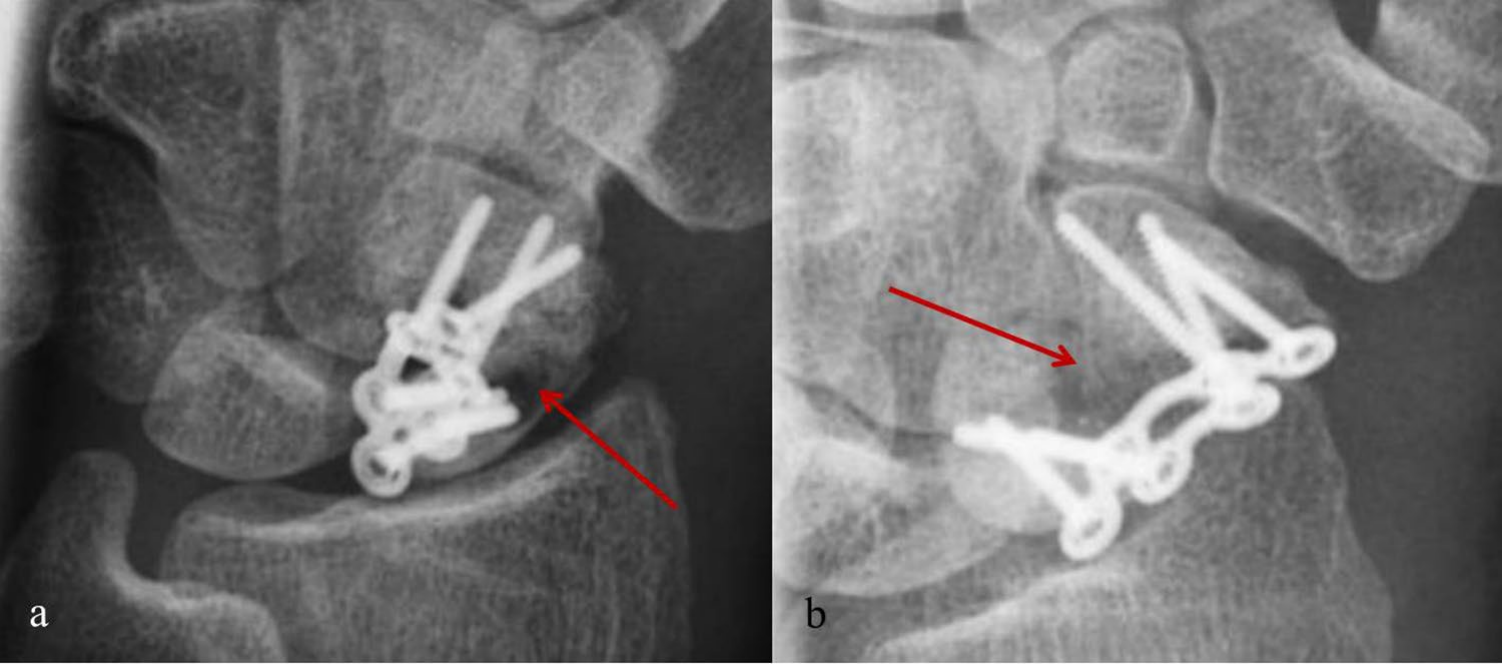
X-ray anteroposterior (a) and oblique (b). Pseudarthrosis in the Scaphoid (arrow) with retained anatomic reposition.
